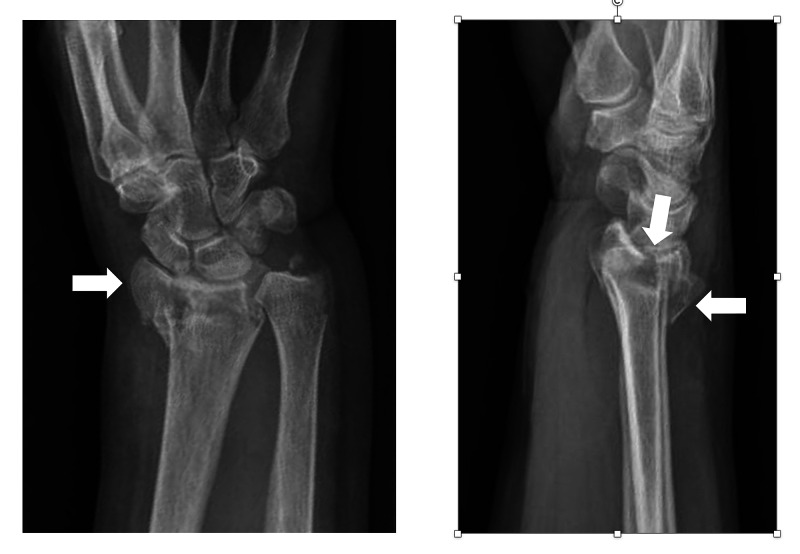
Anteroposterior (AP) and lateral plain radiographs of the right wrist showing initial injury White arrows highlight (from left to right) the separate radial styloid fragment, intra-articular split, and dorsal comminution.
